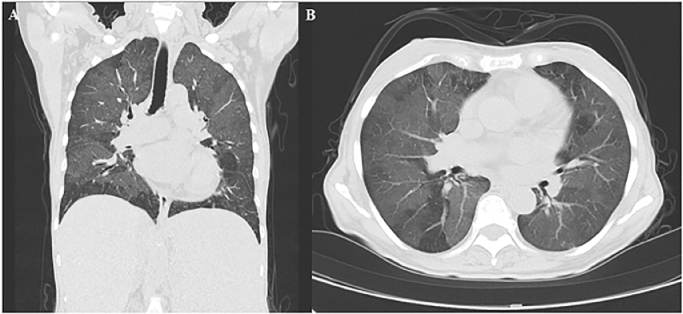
Chest computed tomography images of the patient with pulmonary-extramedullary hematopoiesis at diagnosis. (A) Coronal section and (B) horizontal cross-section. Both images show diffusely extended ground-glass opacity in the lungs.
